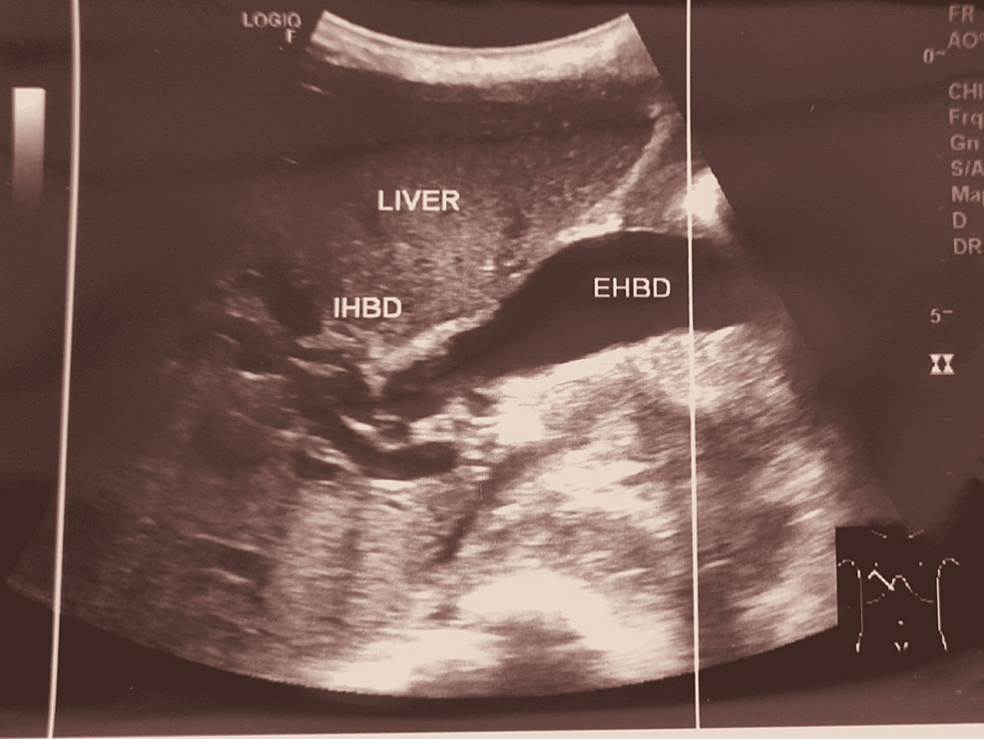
Dilated IHBD and EHBD on abdominal USG. IHBD: intrahepatic biliary duct; EHBD: extrahepatic biliary duct; USG: ultrasonography
